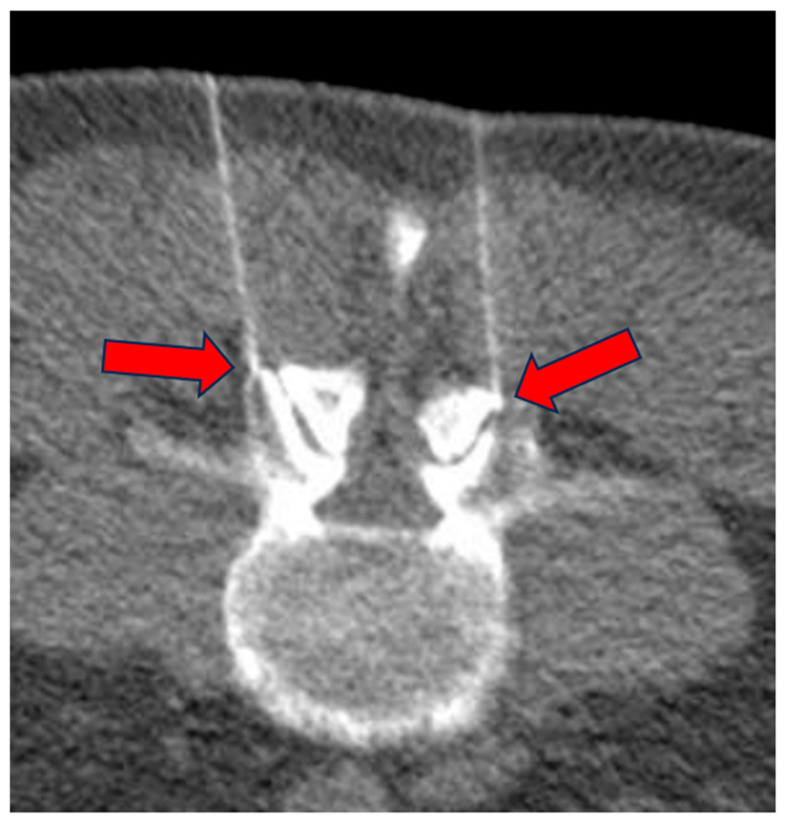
Low-dose CT scan for correct positioning of the needles at the facet joint level (arrows).
